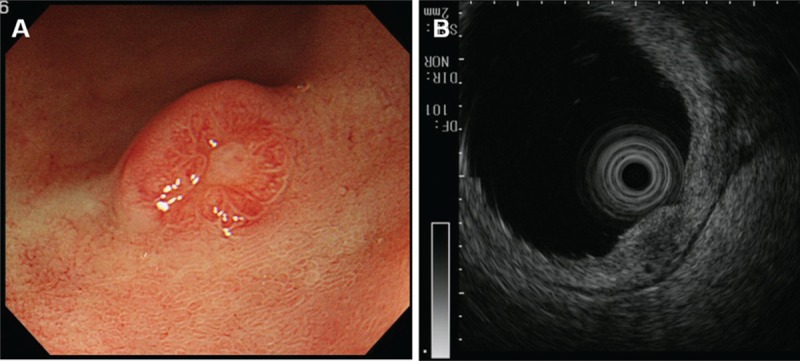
Preoperative examinations by (A) esophagogastroduodenoscopy and (B) endoscopic ultrasonography. Case 1 had a 5.0-mm submucosal NET on the duodenal bulb.
